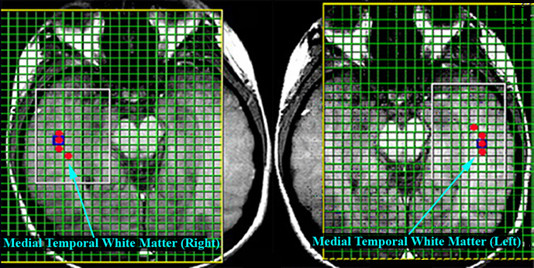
T1-weighted axial slices for left and right MT wm regions with CSI acquisition matrix superimposed as well as ROIs for metabolites quantification.
